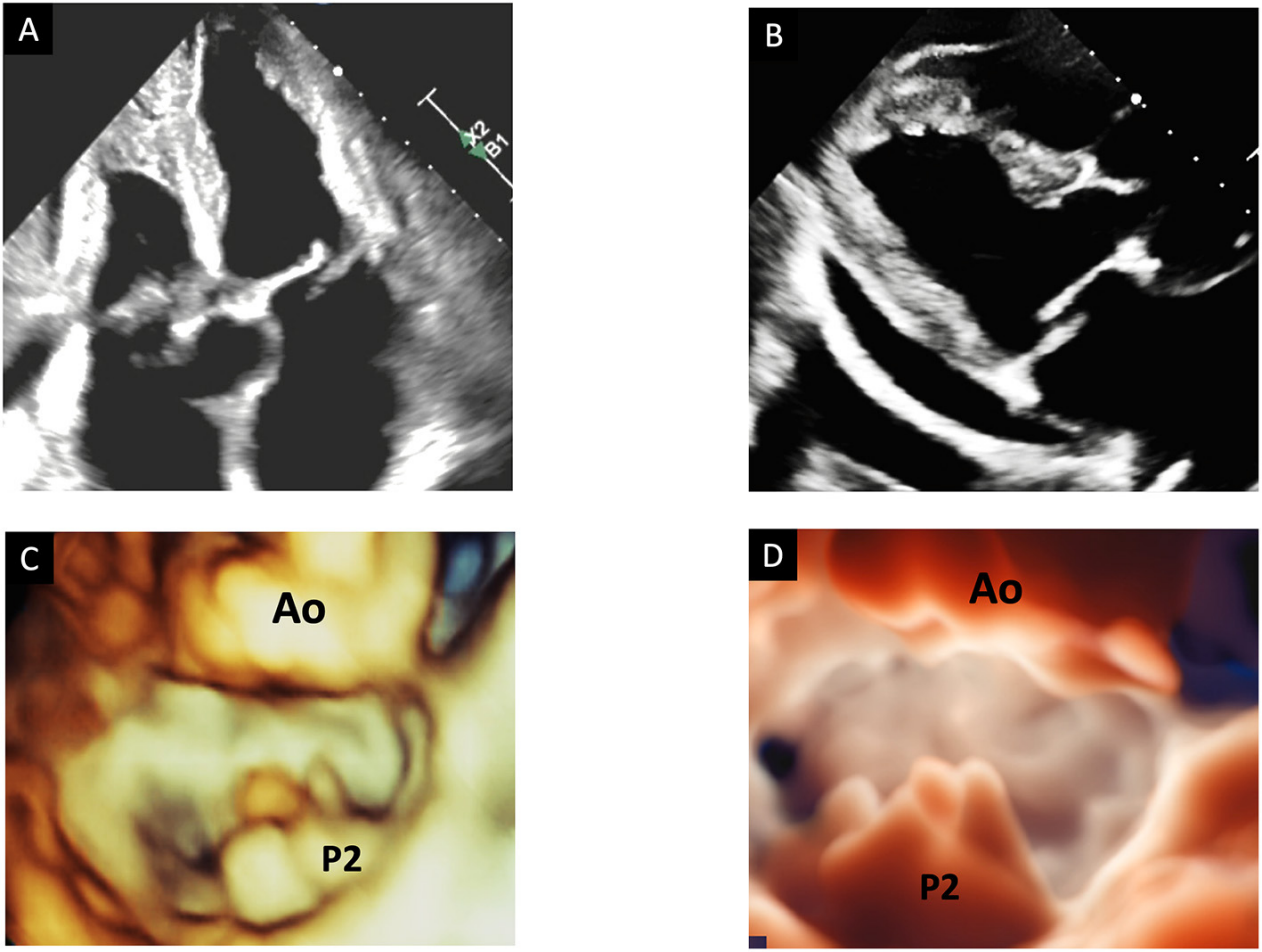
The advantage of transillumination rendering in 3DE. Top panels show an example of P2 flail by 2D TTE with evident eversion of the scallop in a 4-chamber view (A) and in a long-axis view (B). Bottom panels show 3D TTE surgical views of the mitral valve by standard 3D reconstruction (C) and by TI rendering (D). Only TI clearly shows the entire P2 scallop prolapsing in the left atrium with multiple chordal ruptures. 3D, three-dimensional; TEE, transesophageal echocardiography; TI, transillumination. TTE, transthoracic echocardiography.
